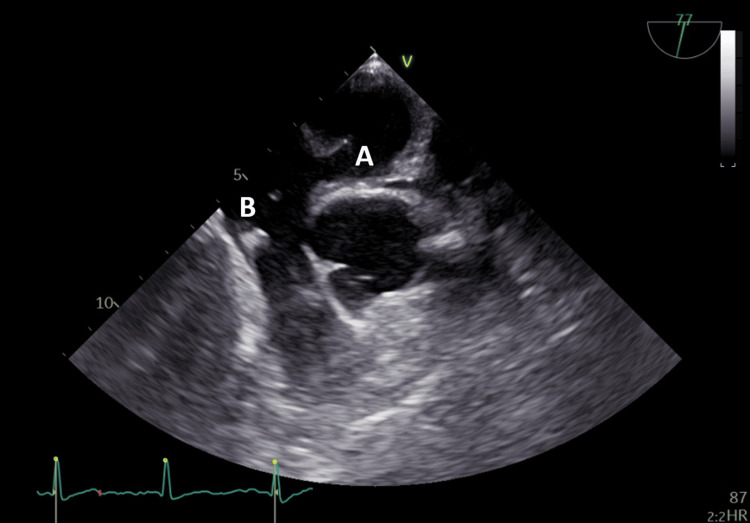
Transesophageal echocardiogram showing patent foramen ovale (A) and Eustachian valve (B).
